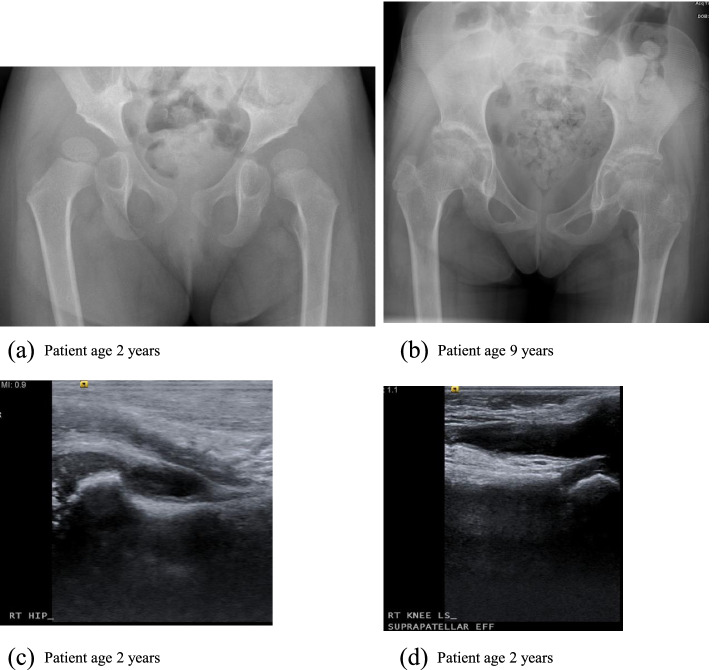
a x-ray pelvis showing mild bilateral acetabular dysplasia with a shallow acetabulum, (b) x-ray pelvis showing short and broad femoral neck with coxa vara and a minimal sclerosis of both acetabular roofs (c) US right hip shows a moderate effusion (d) US right knee shows a moderately large effusion in the suprapatellar pouch
